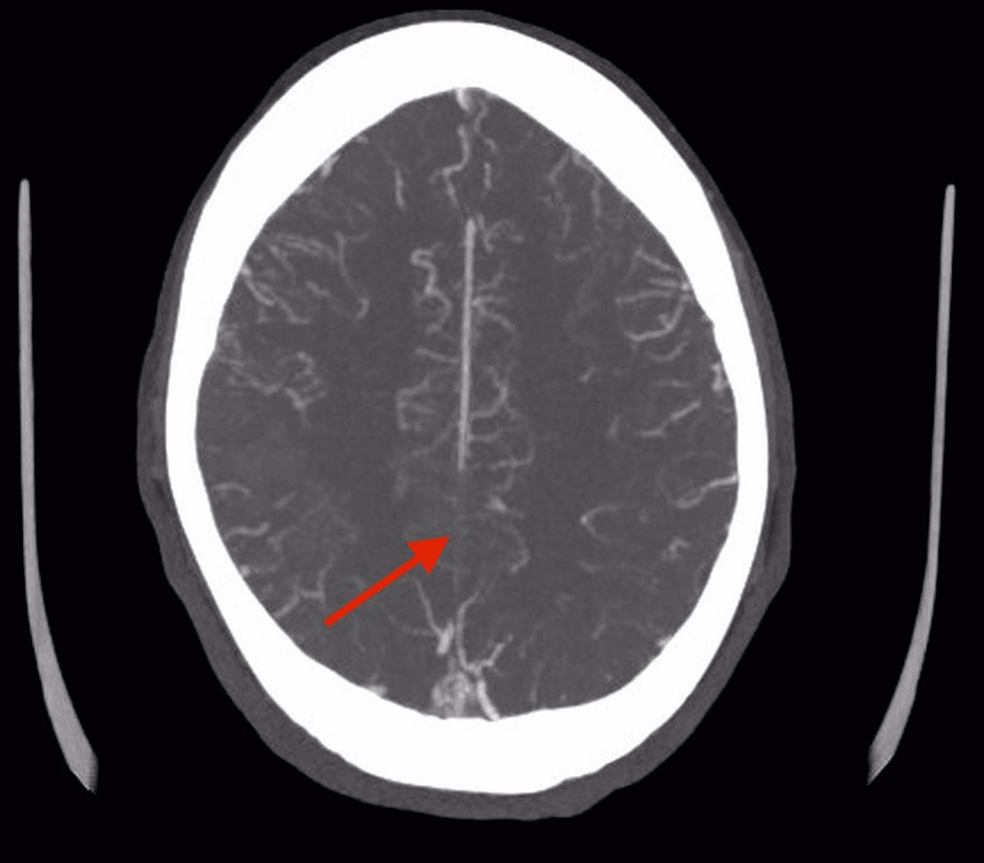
CT venogram of the brain (axial section). The red arrow is pointing toward the superior sagittal sinus where there is no contrast filling indicating a thrombus.
